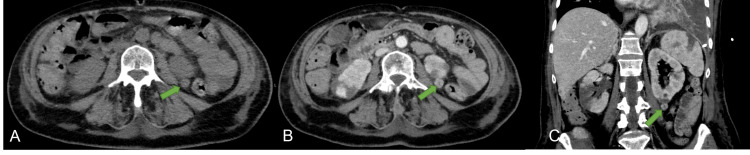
Pre-contrast CT (A) and post-contrast CT (B and C) showing a solid nodule located in the inferior pole of the left kidney (arrows) with contrast enhancement, highly suspicious of a renal neoplasm
